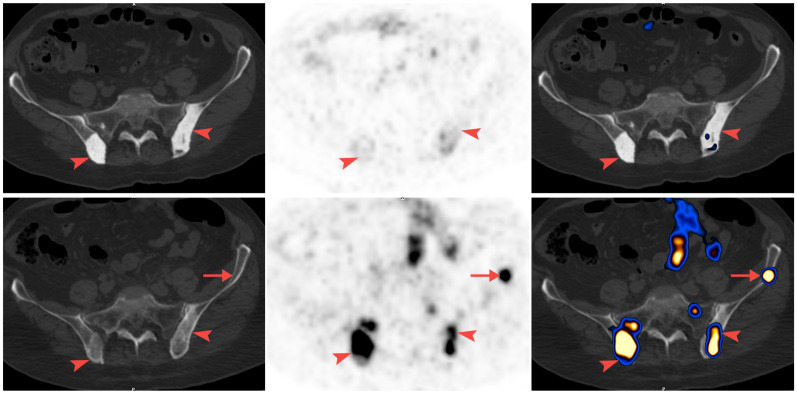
Clinical progression (Patient 14). (From left to right) CT; PET; and fusion images. In the first scan after ADT, sclerotic metastases in the vertebra were identified in pelvic bones with very low uptake of 68Ga-PSMA-11 (arrowhead) (top row). In the second scan, the lesions were no longer sclerotic and showed intense 68Ga-PSMA-11 uptake (bottom row). PSA levels increased and a new metastasis was detected (arrow).
